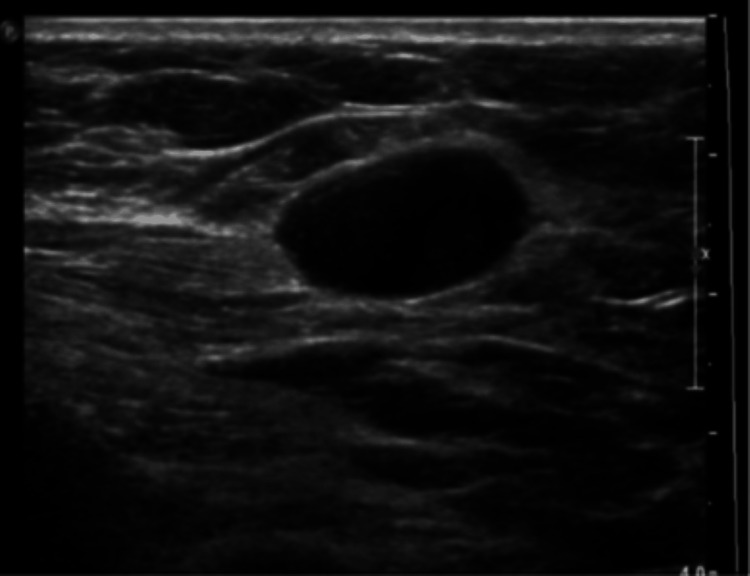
Breast ultrasound BI-RADS: Breast Imaging-Reporting and Data System US BI-RADS 4A lesion was identified.
